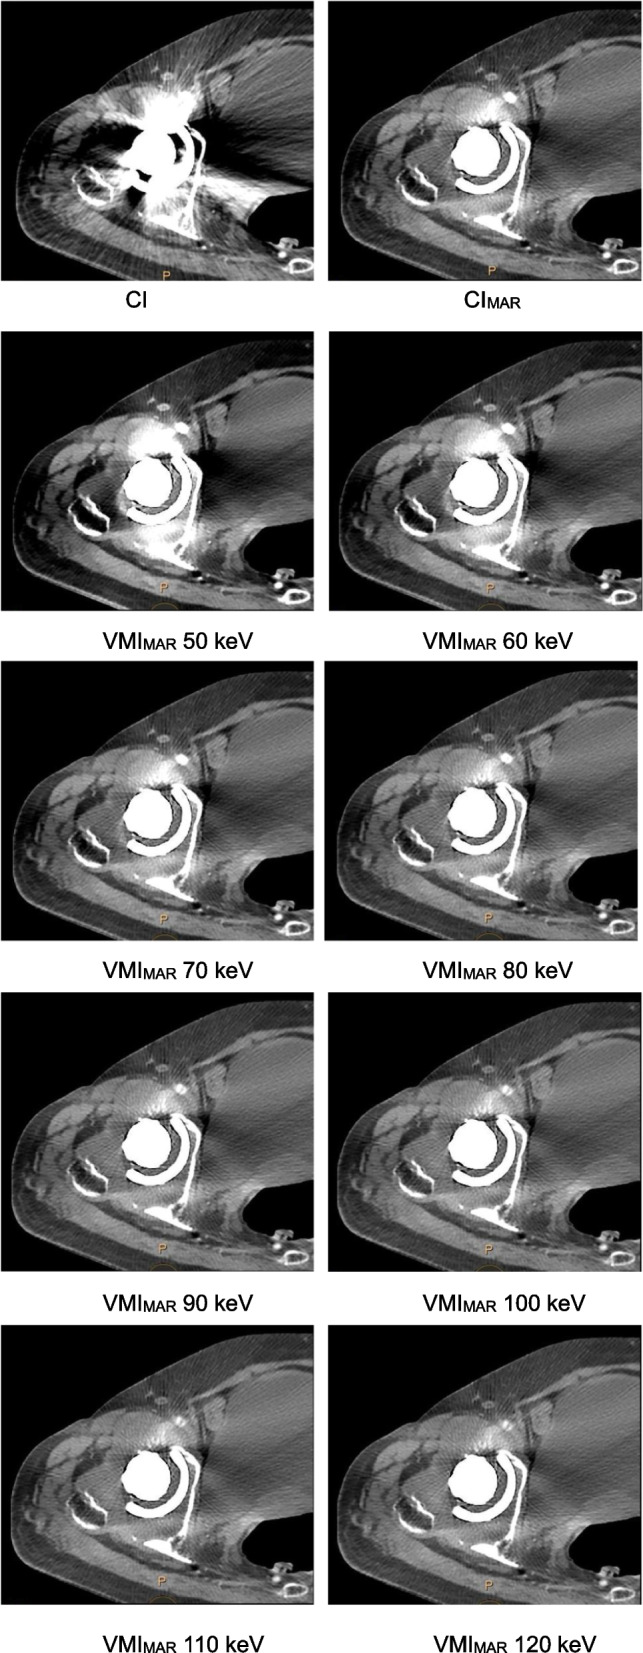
A series of axial enhanced CT images of a patient with THA. Images were reconstructed as CI, CI_MAR_, and VMI_MAR_ at 50–120 keV with 10 intervals (window center 60 HU; window width 360 HU). Images show the metal artifact is gradually reducing with increasing energy level; however, the vascular visualization is worsening. The vascular visualization is terrible above 100 keV, while the vessel is disturbed by artifacts below 70 keV. The best performance is found at 80 keV
